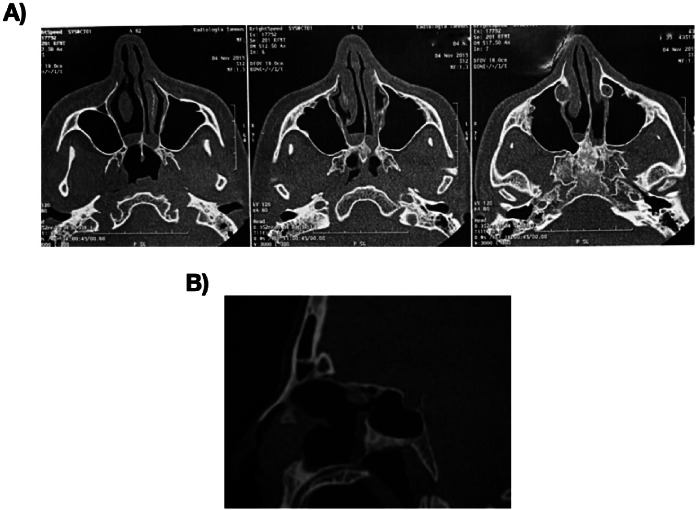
Computed tomography of the facial sinuses showing bilateral osteo-membranous choanal atresia in an axial section (A) and sagittal section (B)
